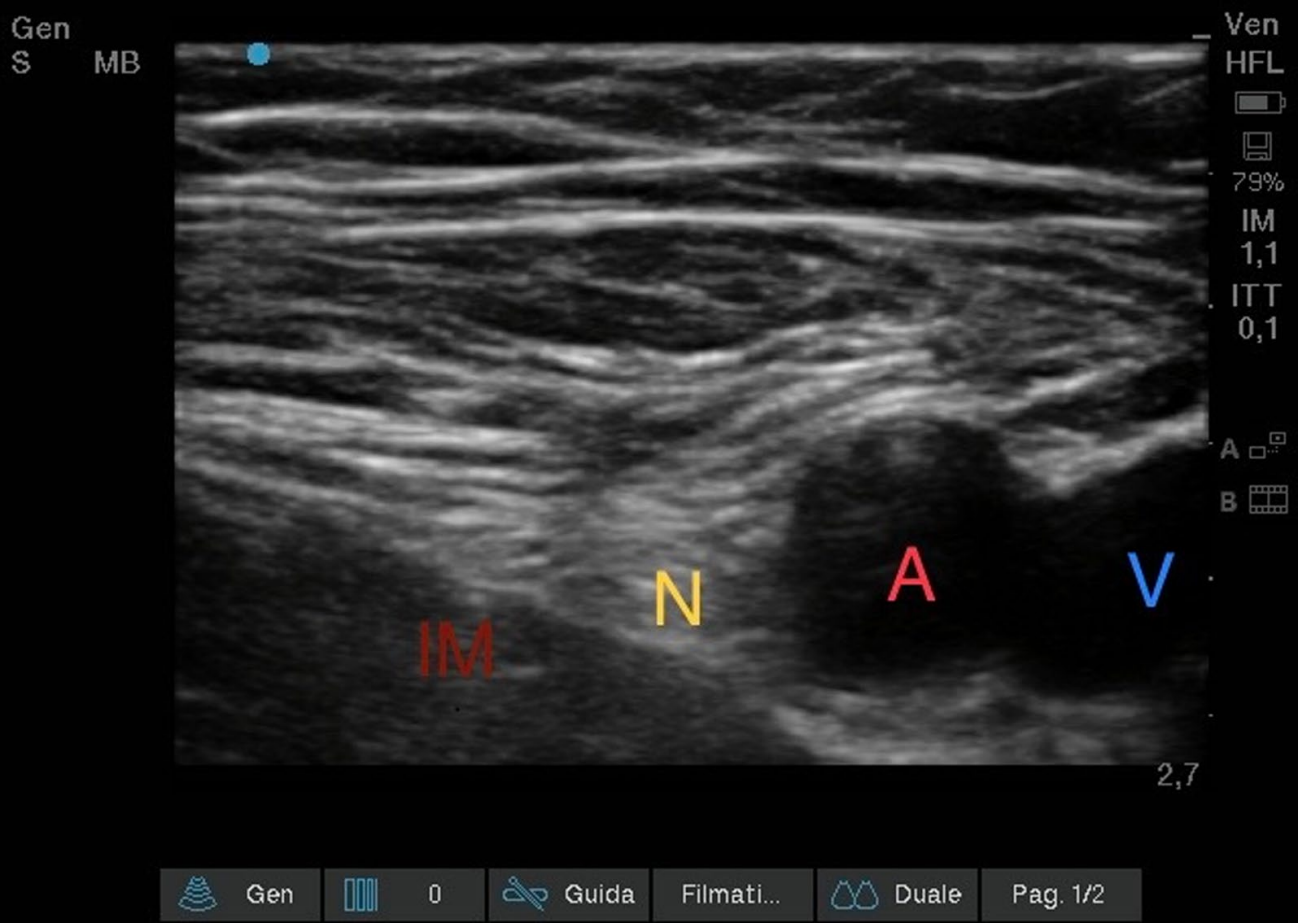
Ultrasound Femoral nerve block. Iliac muscle (IM); Femoral nerve (N); Femoral artery (A); Femoral vein (V)
